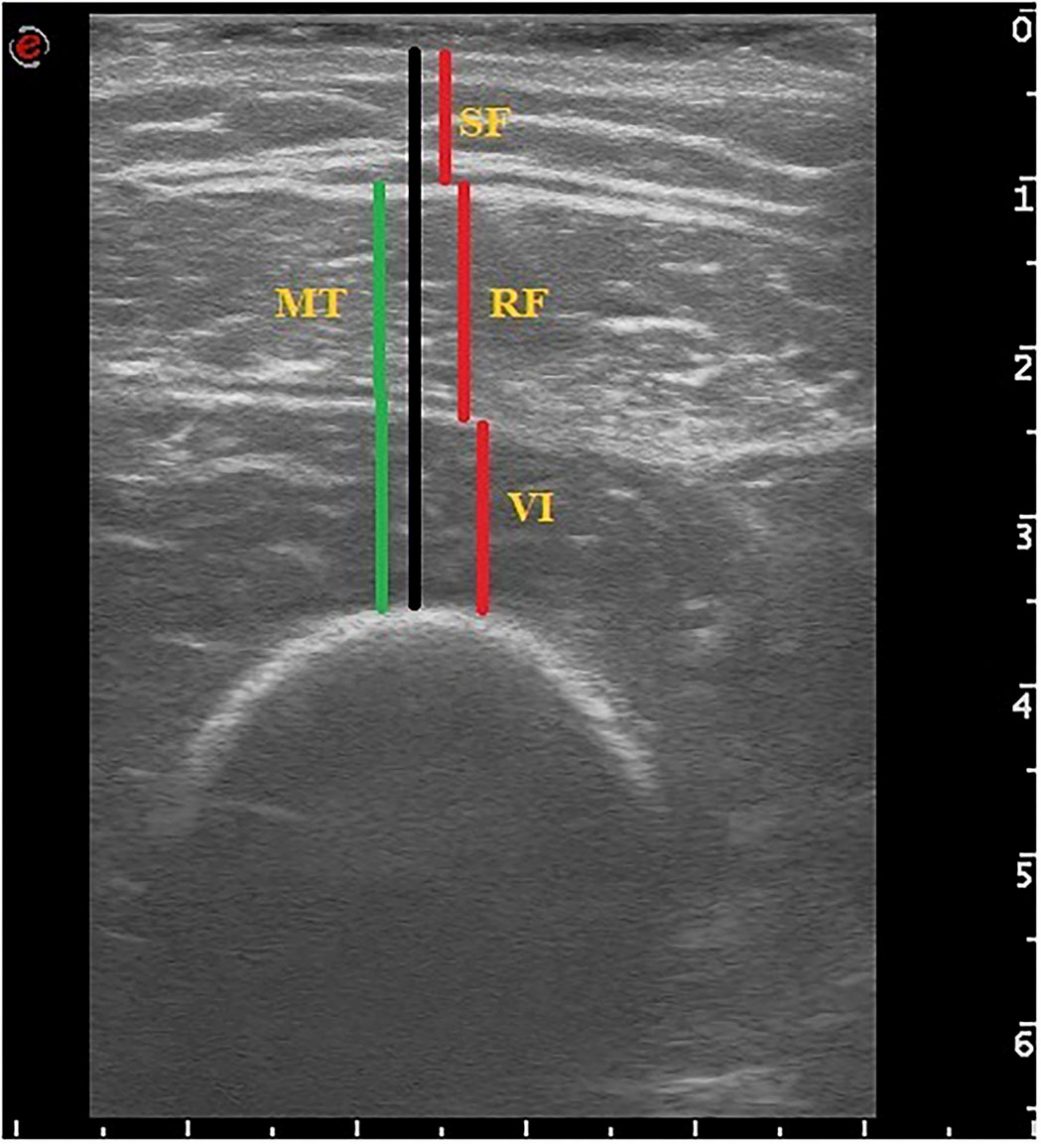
Representative image of muscle ultrasound. Muscle thickness (MT) was defined as the mean value of three measurements of the sum of the distance between the anterior fascia and the posterior fascia of the rectus femoris (RF) and the vastus intermedius (VI) muscles. SF, subcutaneous fat.
